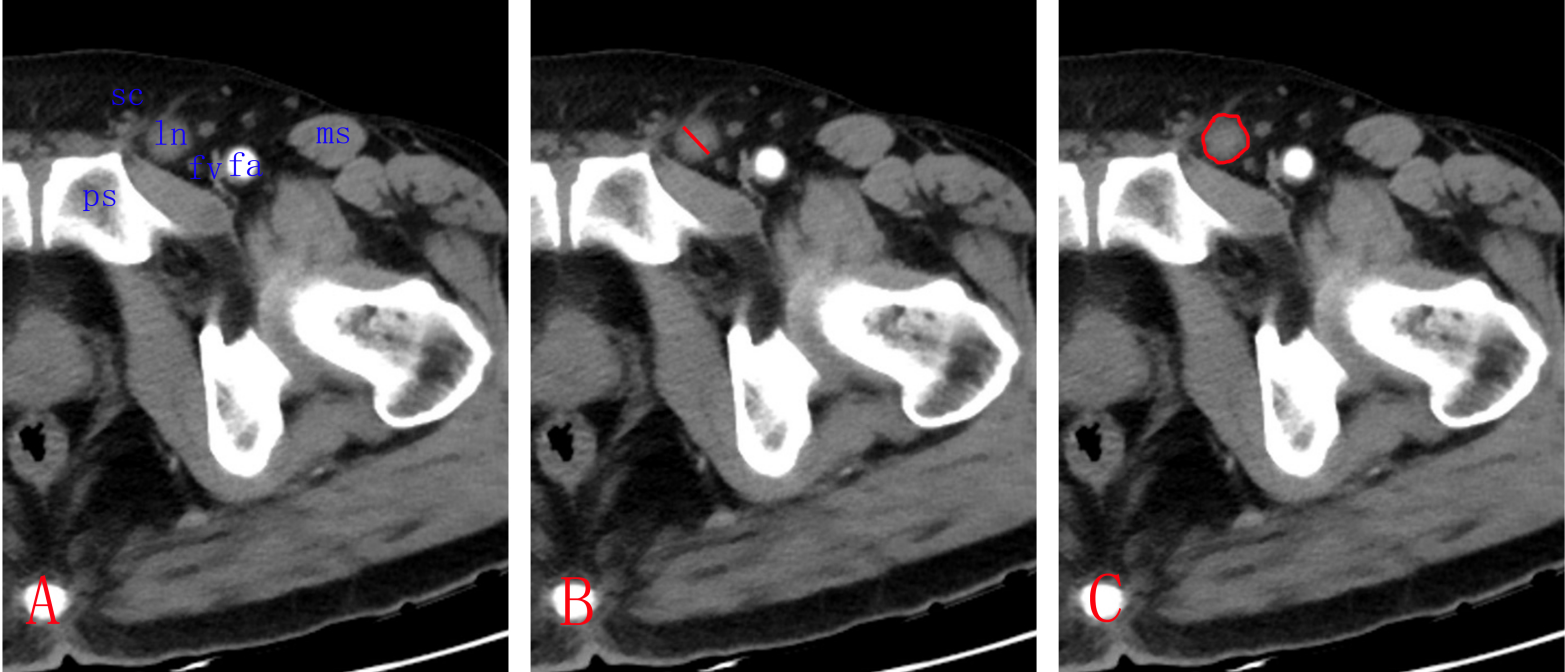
Measurement of inguinal lymph node (ILN) in patients with penile cancer. (A) Choosing the maximum sectioned layer of the target lymph node on the CT image with 5-mm slices. (B) Measuring the short axis diameter of ILN. (C) Tracing the outline of ILN by hand to include the whole lymph node. ln, lymph node; sc, spermatic cord; fv, femoral vein; fa, femoral artery; ps, pubic symphysis; ms, muscle sartorius.
